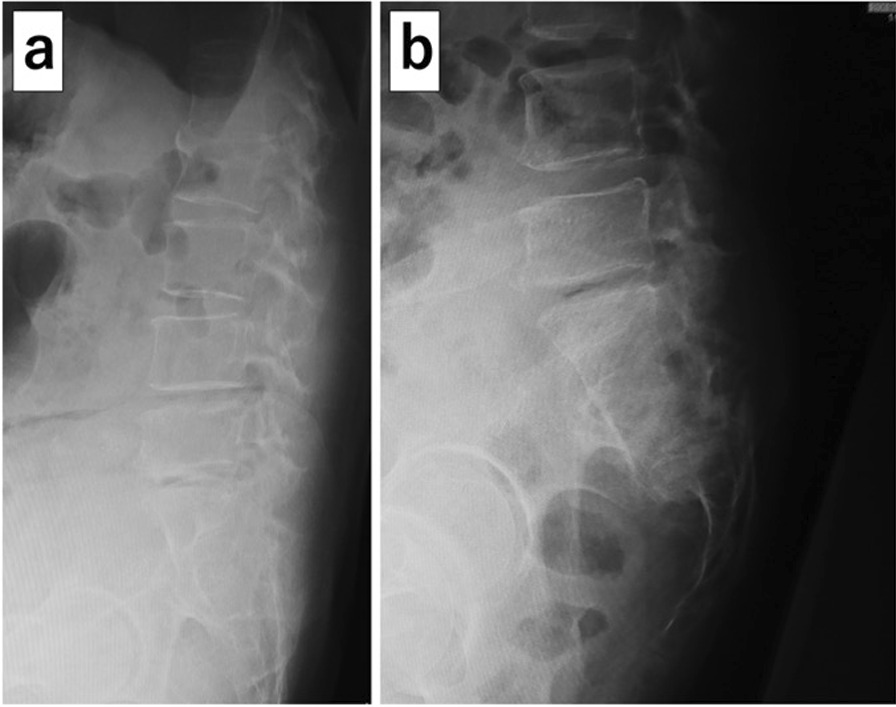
X-ray lateral image. a Lateral image of the lumbar spine on admission to the emergency department. Sacral fracture is not evident. b Lateral image of the sacrum on admission to the orthopedic department. A transverse fracture of the third sacral vertebra with displacement is shown
